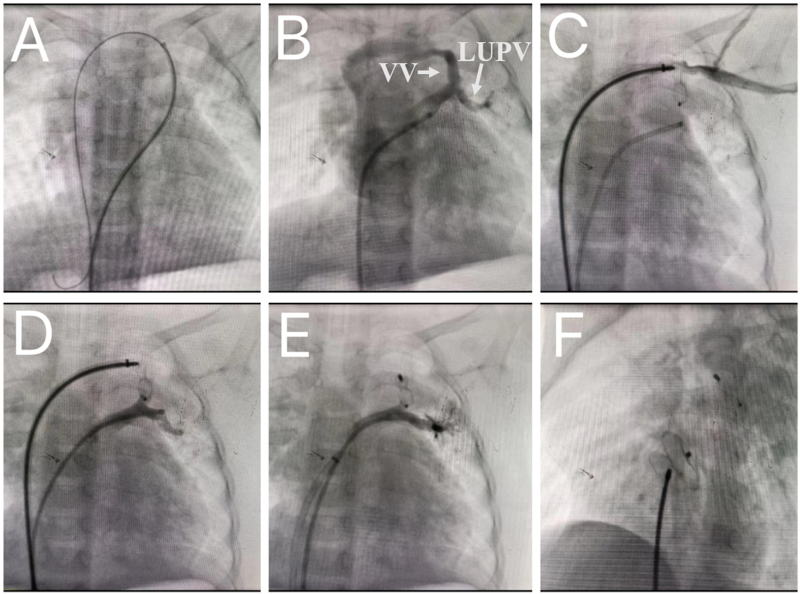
A: The guide wire can pass through the VV, brachiocephalic vein, superior vena cava, and right atrium successively from the left atrium. B: Contrast injection in the LUPV showed that contrast in the LUPV approached the brachiocephalic vein through the VV and flowed into the superior vena cava and the right atrium. C: Left upper pulmonary venography indicates that the LUPV blood returns to the left atrium without obstruction after occlusion. D: Axillary phlebography indicates no obstruction of axillary vein blood flowing back to the left cephalbrachial vein. E: Left upper pulmonary venography indicates that the pulmonary vein flows back to the left atrium. F: Radiography after vertical vein occlusion and atrial septal defect closure. Notes: VV: vertical vein; LUPV: left upper pulmonary vein.
